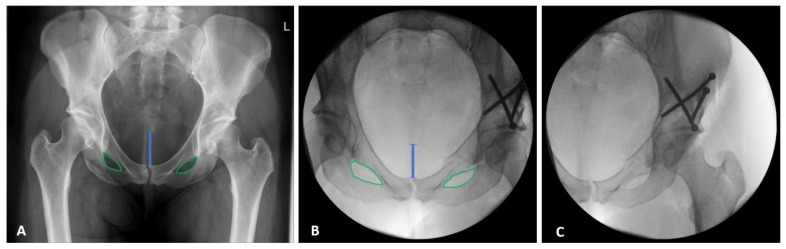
Preoperative radiograph (A) and intraoperative fluoroscopy (B) of the pelvic ring and the obturator foramen (green line) with adjusted tilt, rotation, and coccyx alignment, and with the distance (blue line) from the symphysis to match the preoperative radiograph. Visualized operated hip after lateralization of the C-arm for image acquisition (C).
